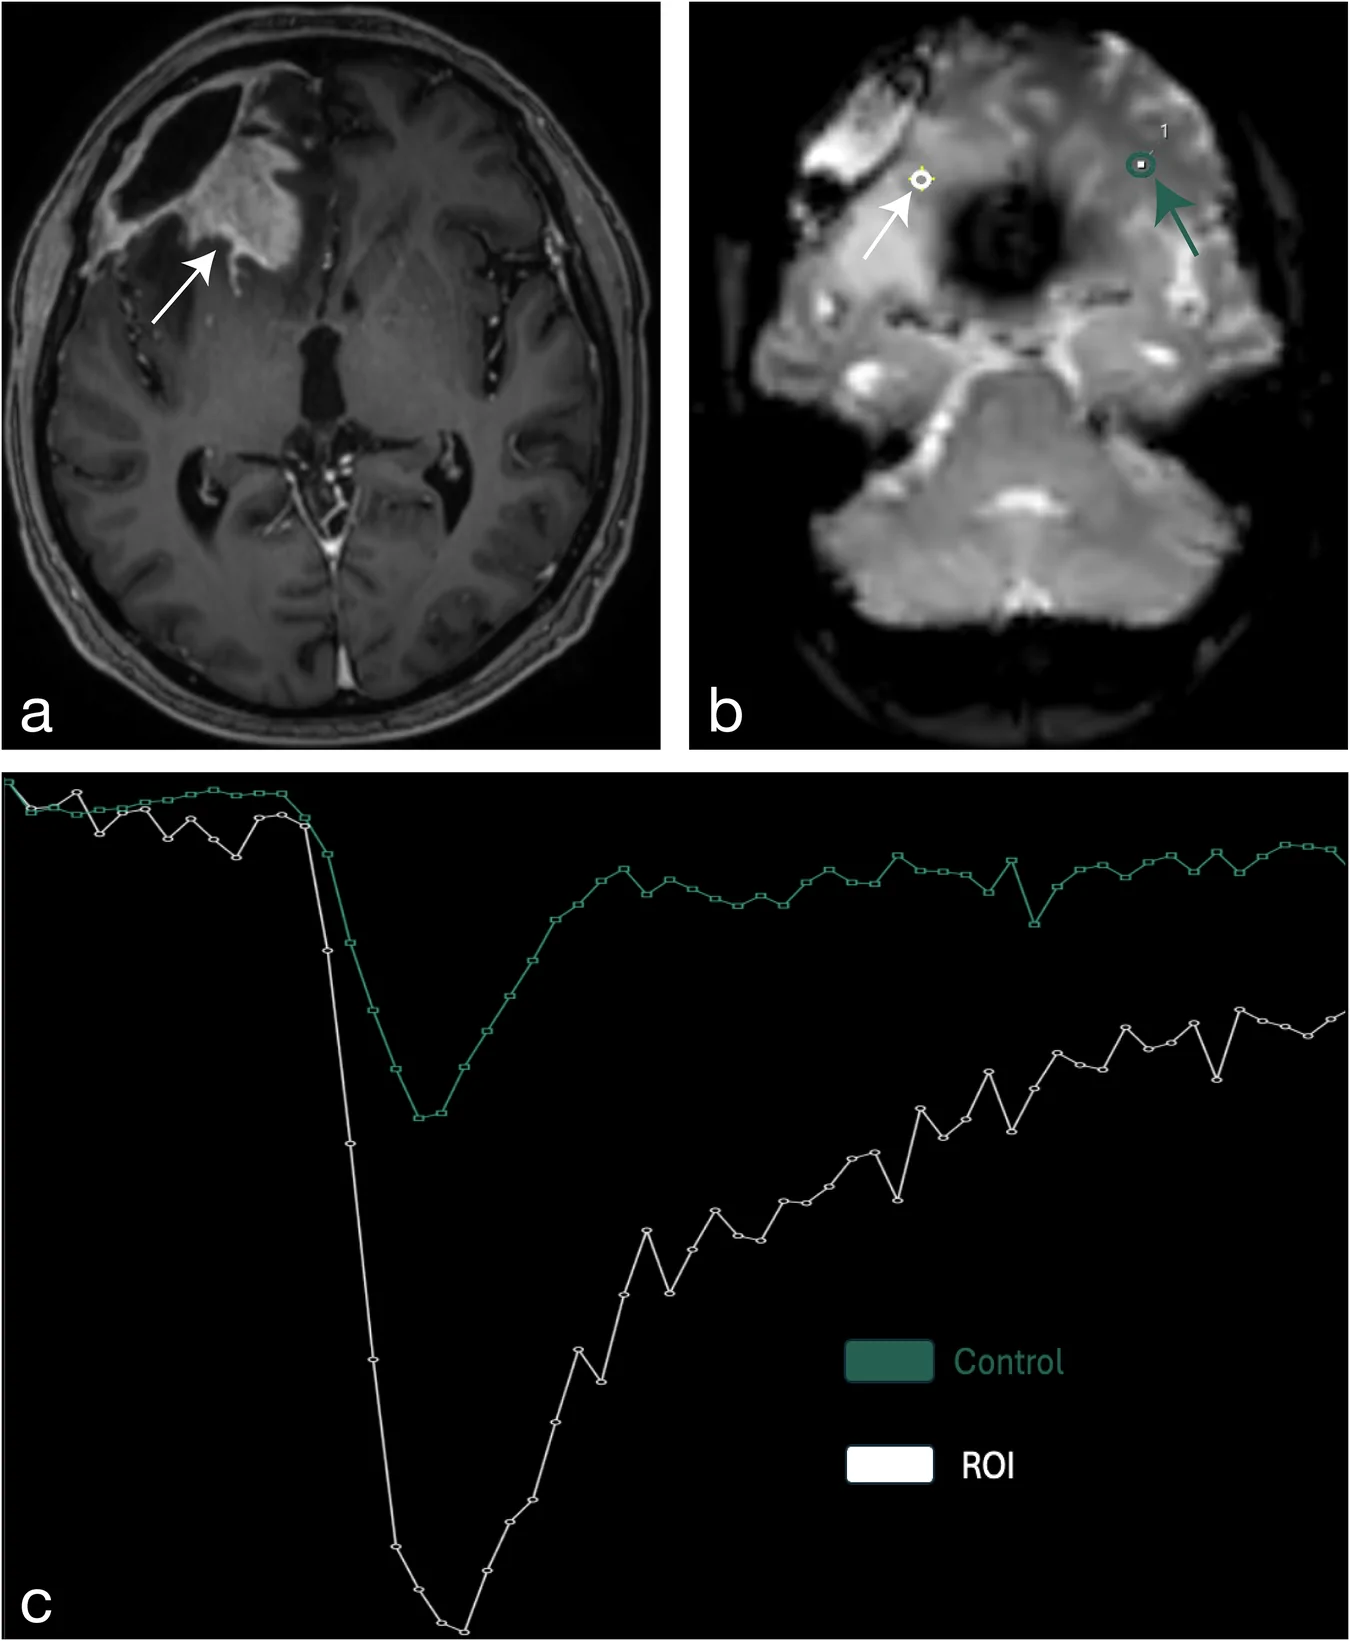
Dynamic susceptibility contrast perfusion in the differentiation of true progression from pseudoprogression. A patient with right frontal glioblastoma scanned in the early post-chemoradiotherapy period, presenting with new contrast-enhancing tissue raising the differential of pseudoprogression versus true tumour progression. a Axial post-contrast T1-weighted image showing a thick irregular enhancing component adjacent to the resection cavity (white arrow). b Dynamic susceptibility contrast (DSC) source image showing the two regions of interest used for perfusion analysis: a lesion ROI placed on the enhancing component (white arrow) and a control ROI placed in contralateral normal-appearing white matter (teal green arrow). c Corresponding time–signal intensity curves: the lesion ROI (white curve) shows a marked signal drop with delayed and reduced post-bolus signal recovery, in contrast to the smaller drop and prompt return to baseline of the control ROI (teal green curve)—a pattern indicating elevated relative cerebral blood volume (rCBV) with low percentage of signal recovery (PSR) in the lesion, favouring true tumour progression over pseudoprogression
